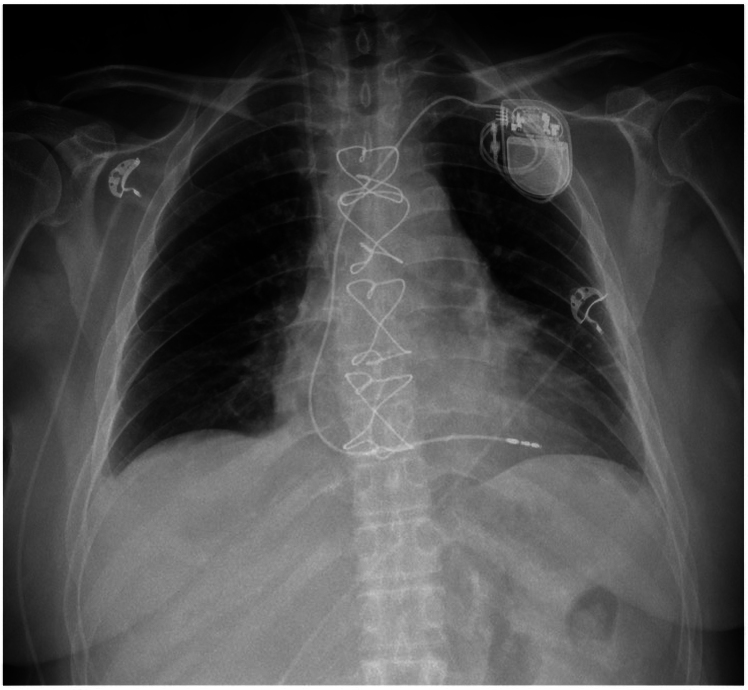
Anterior posterior (AP) chest X-ray confirmed the pacemaker and lead position. Own study.
